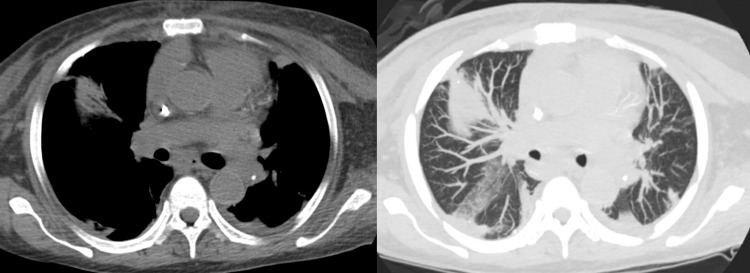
(Left) Noncontrast axial CT demonstrating left pulmonary artery embolism with cast visualized in the superior vena cava. (Right) Maximum intensity projection view demonstrates same findings.
